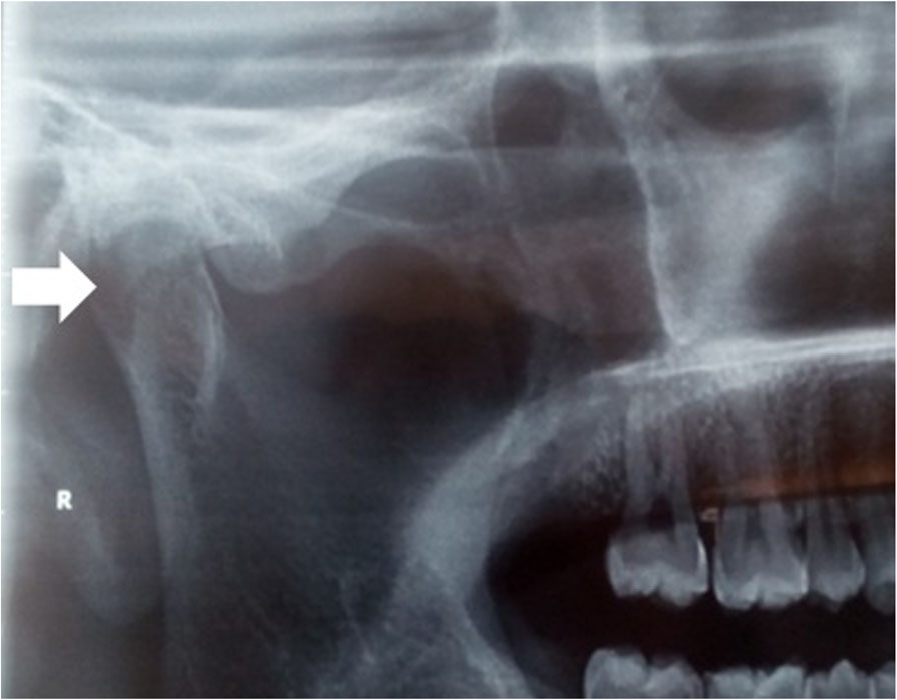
X-ray image showing a normal temporo-mandibular joint (arrow head)
